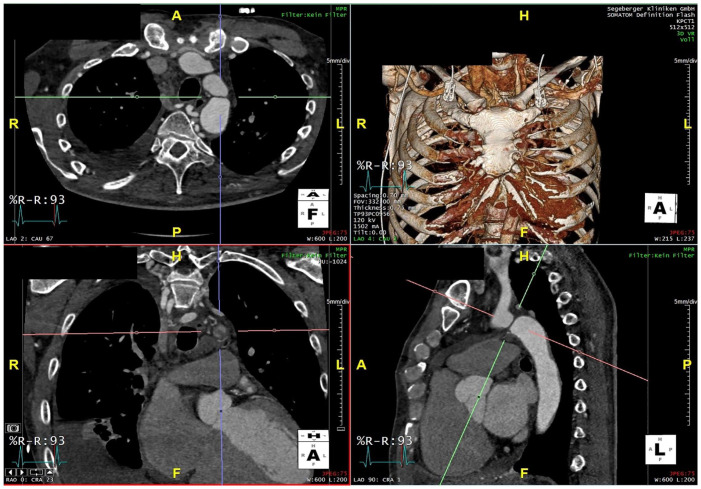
Preoperative computed tomography angiography (CTA) with aortic coarctation.
